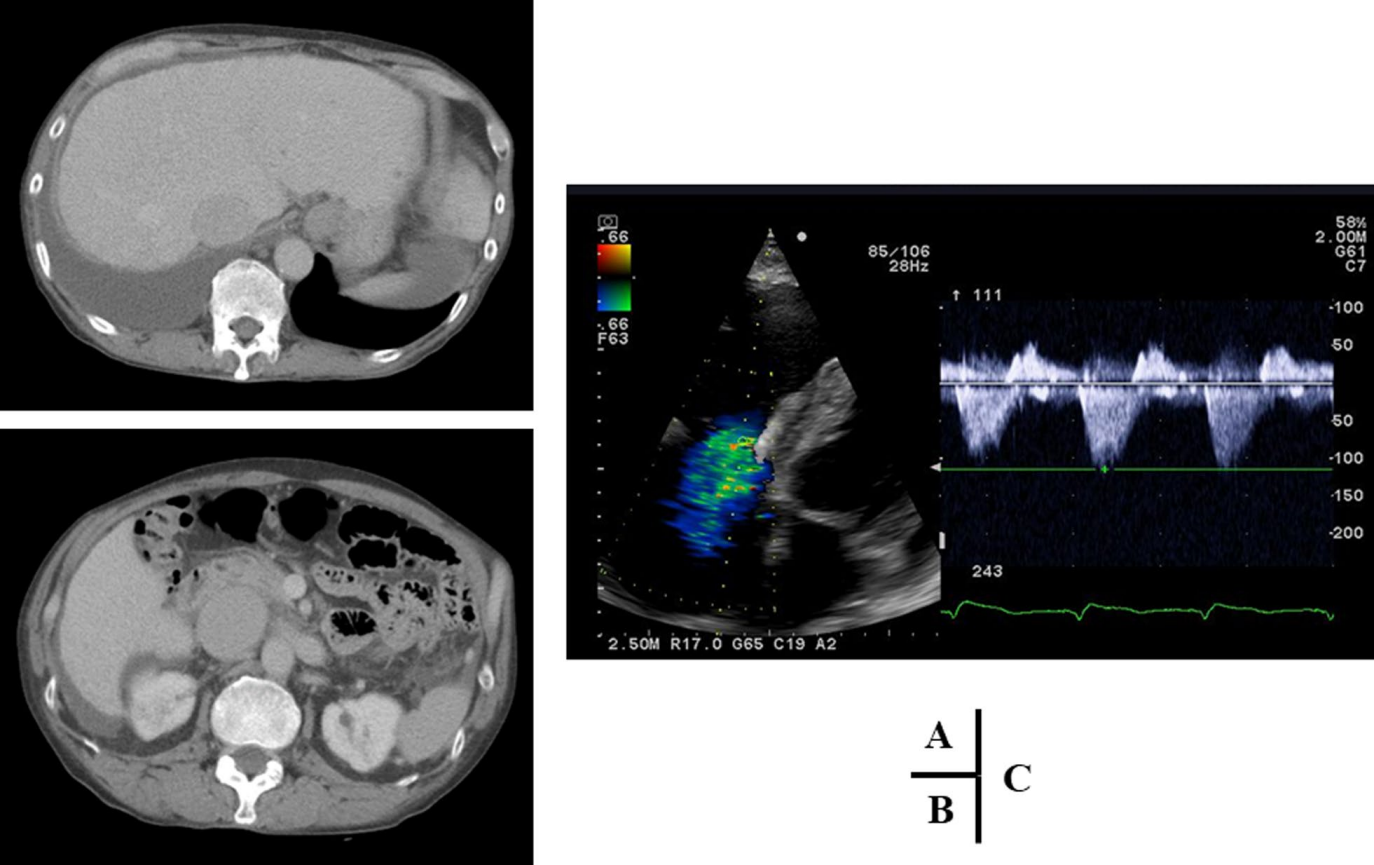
Computed tomography revealed the presence of congestive liver and inferior vena cava dilatation (A, B). Echocardiography showed prominent enlargement of the right atrium and right ventricle, and severe TVR (C)
